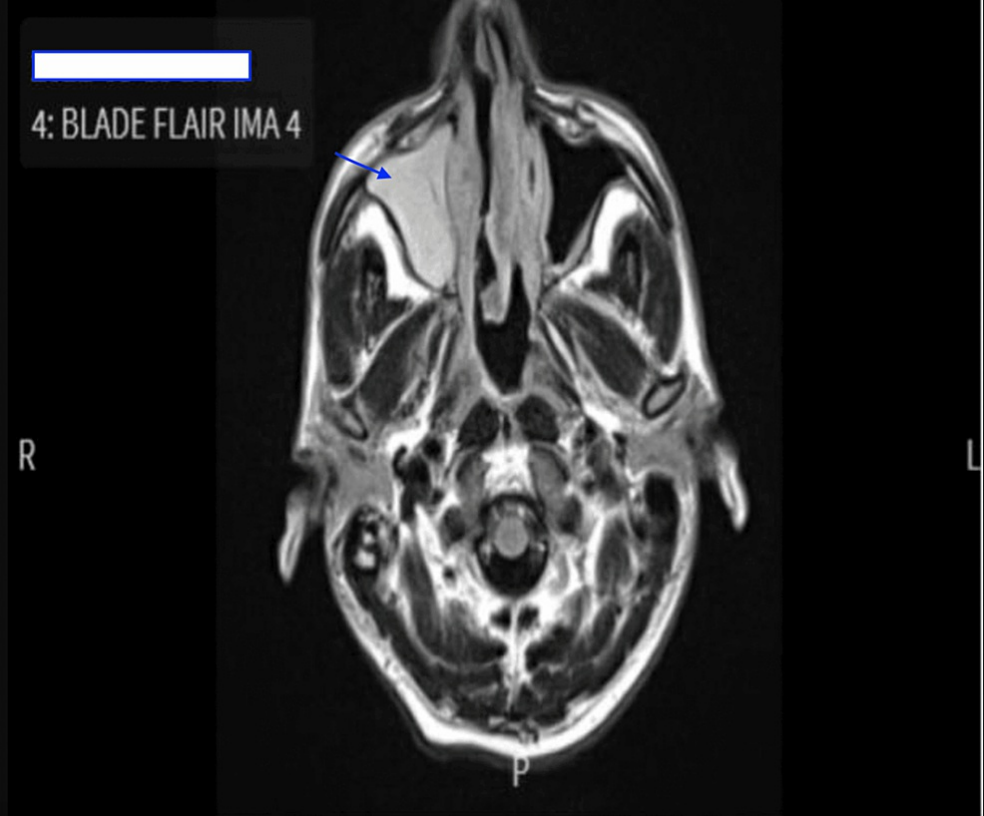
MRI brain with contrast showing necrosis of the soft tissues in the right nasal cavity.
